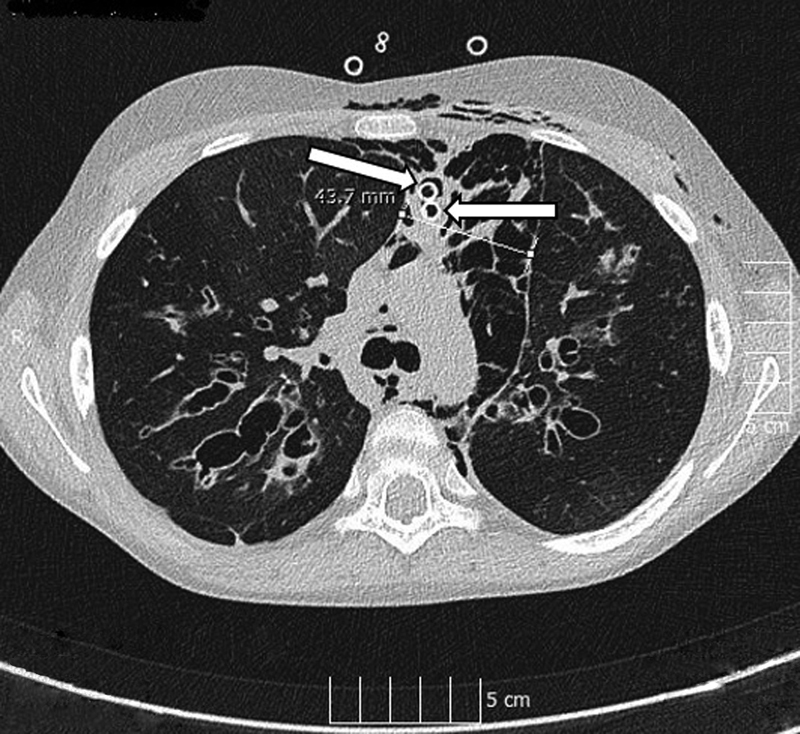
Control computed tomography scan (after 11 days of the first one) depicted a significant improvement (4.3 cm). The arrows show the mediastinal drains.
